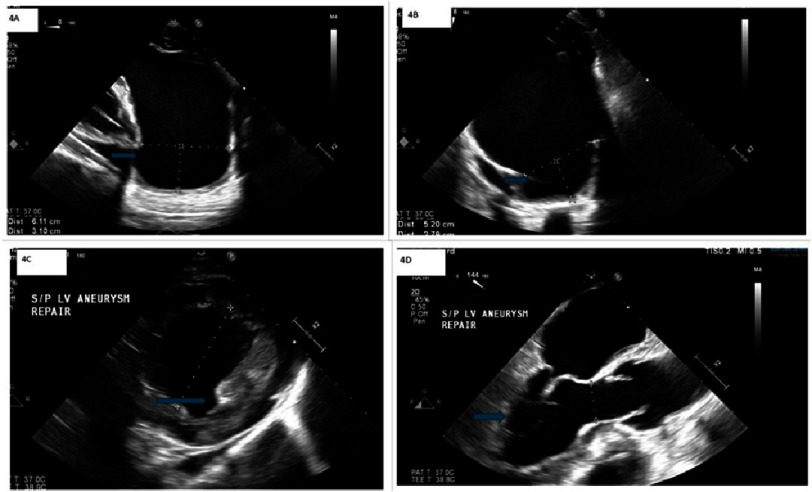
Intraoperative transesophageal echocardiography (TEE) shows a 6.3 cm left ventricular apical aneurysm (4A, 4B), with hypokinesis of the basal wall and akinesis of the remaining walls, resulting in an ejection fraction of 5 to 10 percent. Post-surgical repair TEE (4C, 4D) demonstrates normal left ventricular size, with moderate hypokinesis of the lateral and inferior walls extending to the papillary area, and akinesis of the remaining walls. The ejection fraction is now measured at 15–20 percent.
